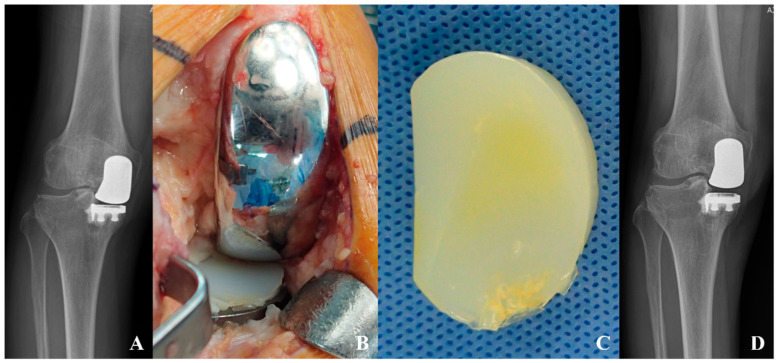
Isolated polyethylene insert exchange for polyethylene wear after medial fixed-bearing unicompartmental knee arthroplasty (UKA). A 57-year-old female patient who underwent medial fixed-bearing UKA in the right knee. The patient remained asymptomatic for 8 years postoperatively but developed vague pain on knee bending that persisted for 2 years. (A) Plain radiographs showing slight varus alignment with asymmetric narrowing between femoral and tibial components. (B,C) Intraoperative findings demonstrating polyethylene wear on the anteromedial aspect. Both femoral and tibial components remained stable and well-fixed. (D) Postoperative radiographs after isolated polyethylene insert exchange performed at 9.8 years after the initial surgery.
